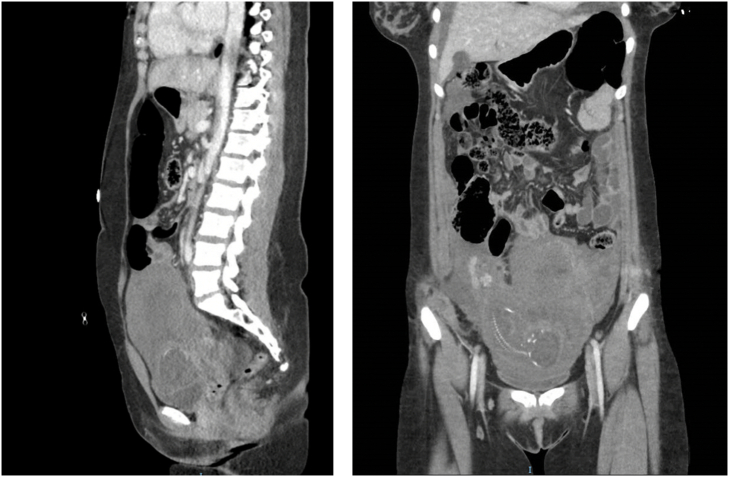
CT abdomen/pelvis in sagittal view demonstrating hemoperitoneum and unclear uterine boundaries in the sagittal (left) and coronal (right) views.
